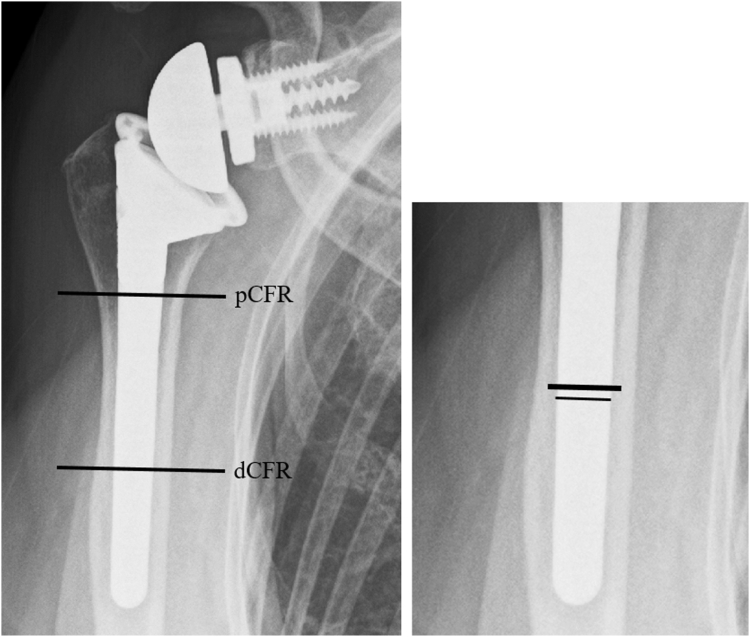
The canal filling ratio was calculated at the proximal and distal third of the humerus using plain radiographs immediately after surgery.
